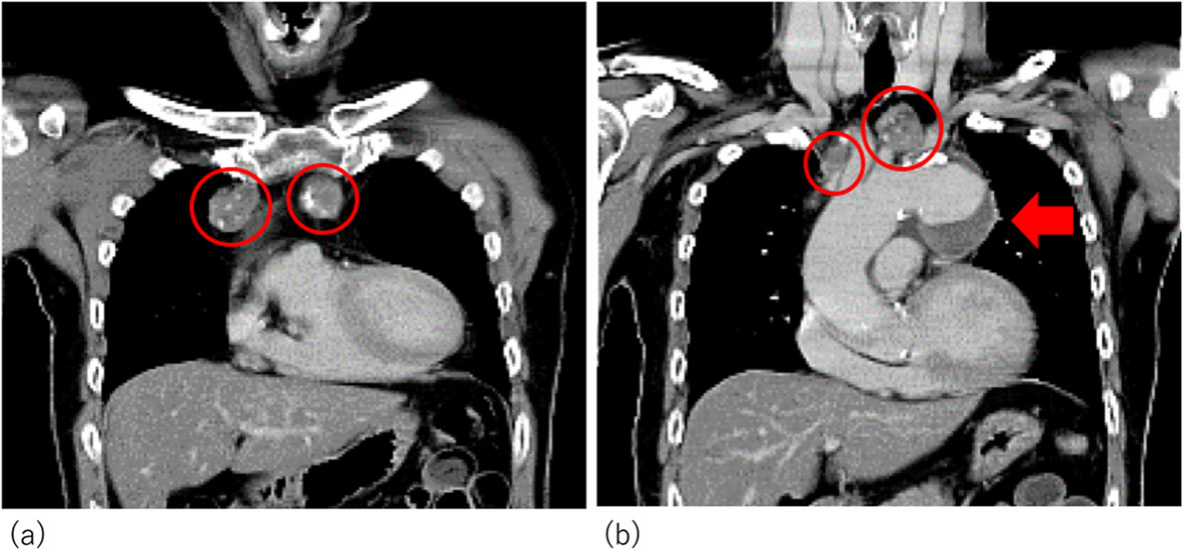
Computed tomography revealed four lesions in the anterior mediastinum (circles) and an aortic aneurysm (arrow)
